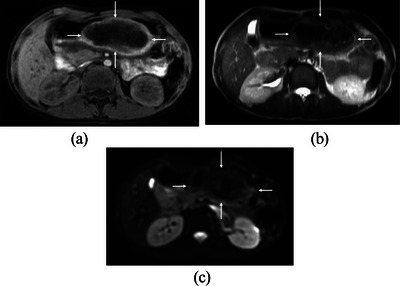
Magnetic resonance imaging. T1‐ (a), T2‐ (b), and diffusion‐weighted (c) images showed a hypo‐intense pattern of the mass.
